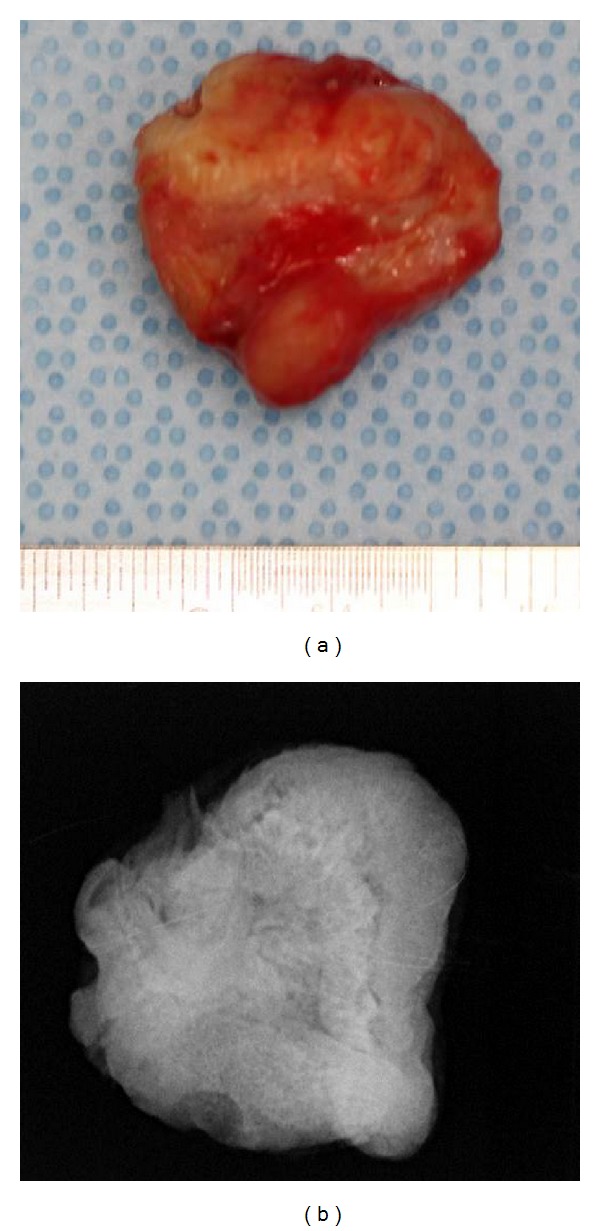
View of the excised lesion. (a) Macroscopic findings. (b) X-ray finding.
